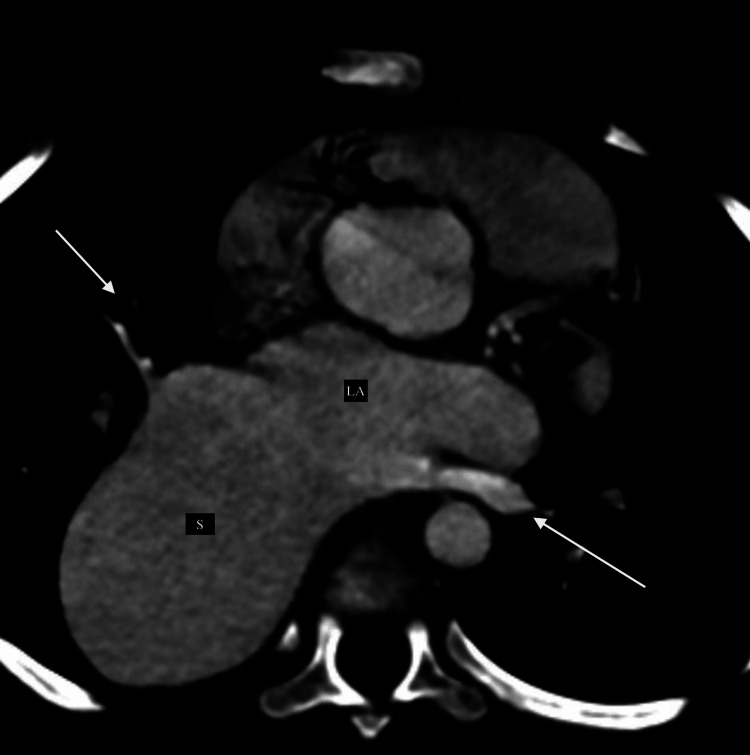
Axial CT reformatted images showing the normal drainage of pulmonary veins into the left atrium (shown by arrows). S: dilated aneurysmal sac: LA: left atrium
